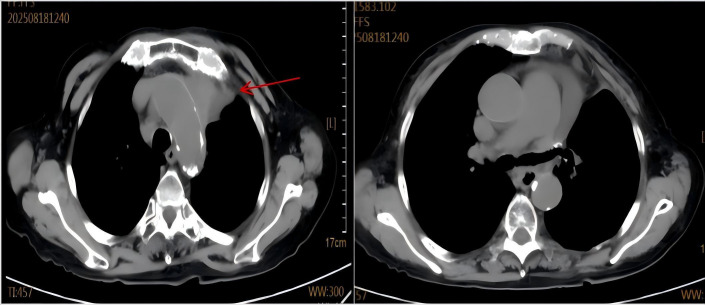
The August 2025 follow-up chest CT shows a marked reduction in the mediastinal tumor mass (red arrow) and resolution of the pericardial effusion.
